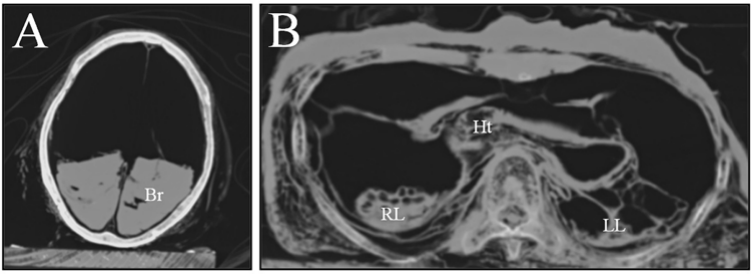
The CT images of this case. (A) for head; (B) for thorax. Most mummified organs were displaced to the dorsal side of body cavities. Their morphologies were seriously deformed. Br, brain; Ht, heart; RL, right lung; LL, left lung.
